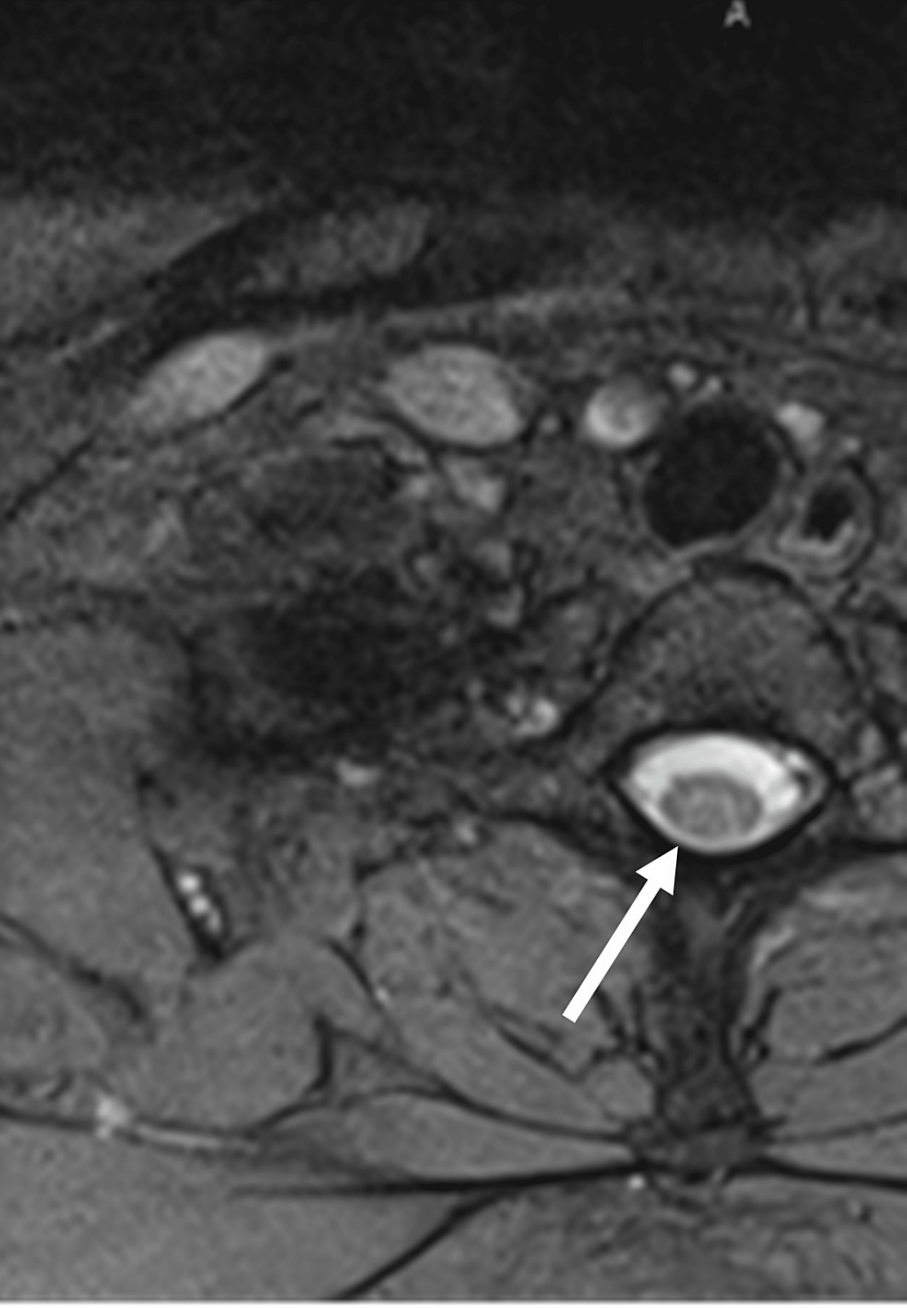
MRI of the cervical spine revealed an abnormal T2-hyperintense signal involving the dorsal column of the spinal cord.
